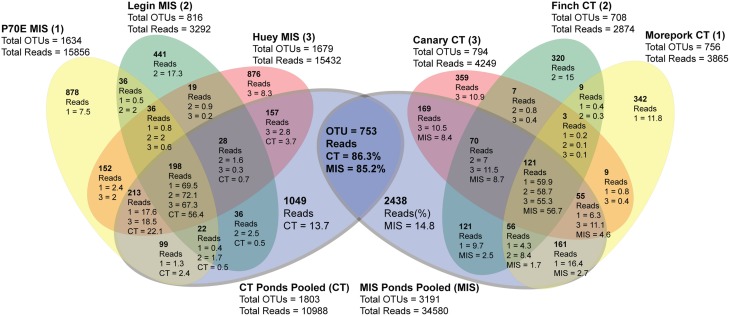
Summary of 454 sequencing data (distance = 0.05) representing shared OTUs (in bold) and reads (%) from individual ponds and pooled data between sample locations. MIS ponds pooled (MIS) represents all P70E, Legin and Huey reads to compare with individual CT ponds; CT ponds pooled represents all Morepork, Finch and Canary reads to compare with individual MIS ponds.
